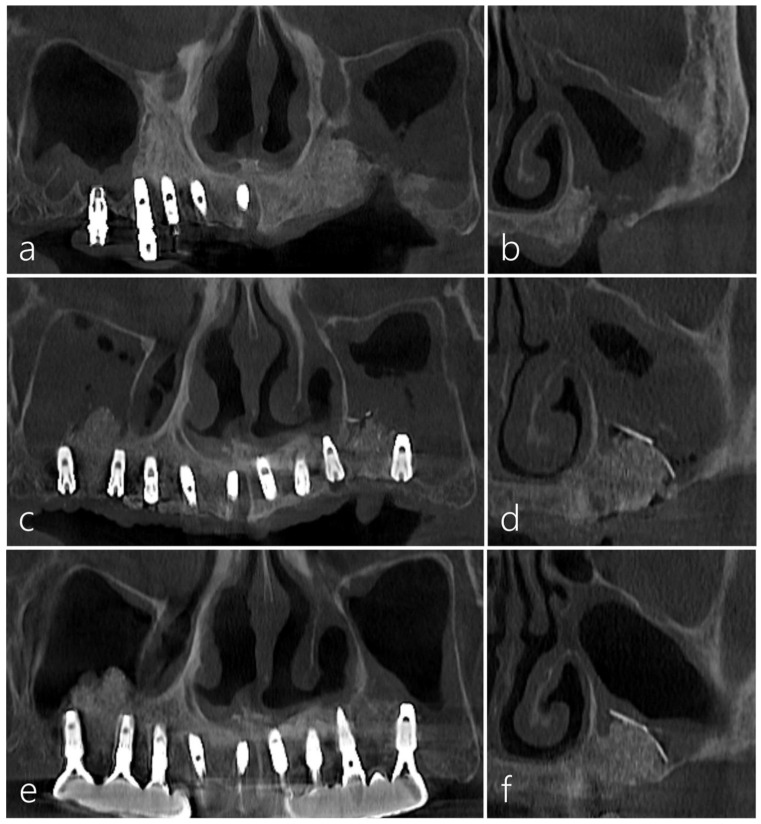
(a) The preoperative CBCT image showed a significant loss in the augmented bone graft in the left maxillary posterior area. (b) Sinus mucosal thickening was observed in the left maxillary sinus. (c,d) The augmented bone graft and titanium-reinforced d-PTFE membrane were observed in the panoramic and coronal CBCT scans taken immediately after the procedure. (e) In the CBCT image taken 12 months after the final prosthesis was delivered, reduced sinus mucosal thickening was observed. (f) The coronal CBCT image showed the well-maintained titanium-reinforced d-PTFE membrane on the right maxillary sinus.
